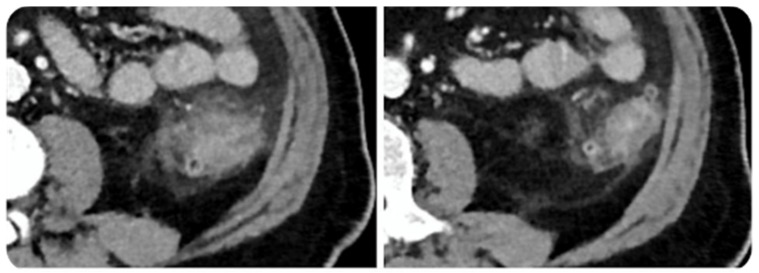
Complicated immune-related segmental colitis with diverticulosis pattern in a 76-year-old man with angiosarcoma and abdominal pain during Nivolumab treatment. CT images of two different slices show a segmental and circumferential descending colon wall thickening and pericolic fat stranding. © Department of Radiology, Candiolo Cancer Institute—FPO-IRCCs. Turin.
